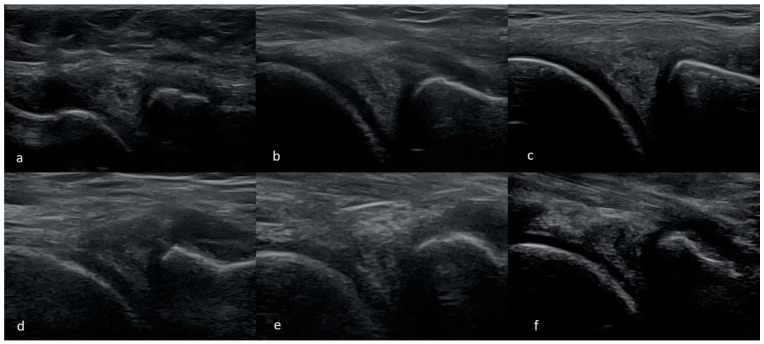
Images of intrasubsantional damages of menisci (a) corresponding to type I lesion seen in MRI; (b,c) to type II; (d–f) to type III.
